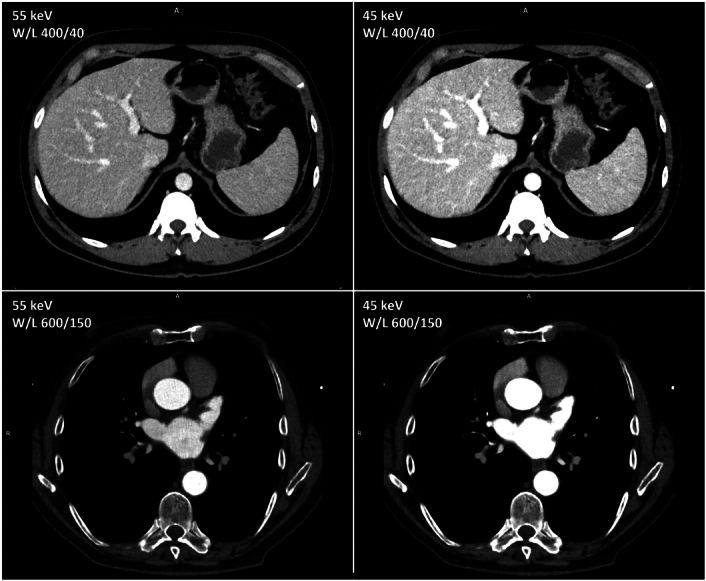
Example images of an abdomen PVP (upper row) and CTA (bottom row) scan reconstructed at 55 keV (left) and 45 keV (right). The BMI of the abdomen PVP patient was 27.8 kg/m^2^, and of the CTA patient 25 kg/m^2^. CNR increased from 4.18 to 5.04 (+20.6%) for the abdomen PVP, and from 20.6 to 26.1 (+27%) for the CTA, for the 10 keV decrease in VMI energy.
